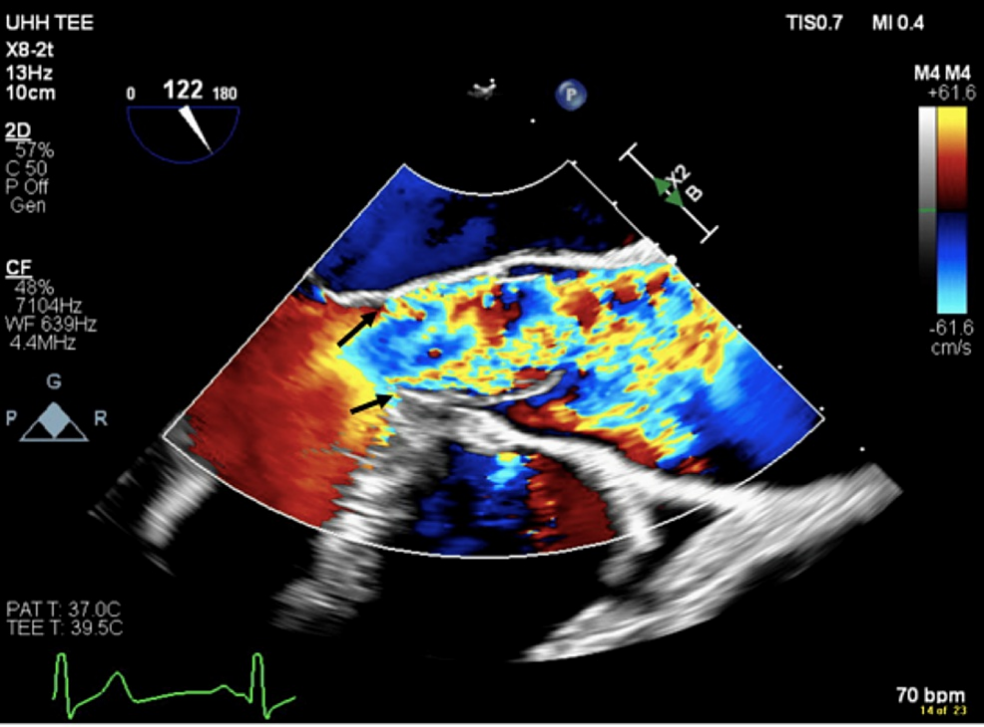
Transesophageal echocardiogram with and without color Doppler demonstrating the presence of a subaortic membrane causing regurgitant blood flow through the aortic valve.
